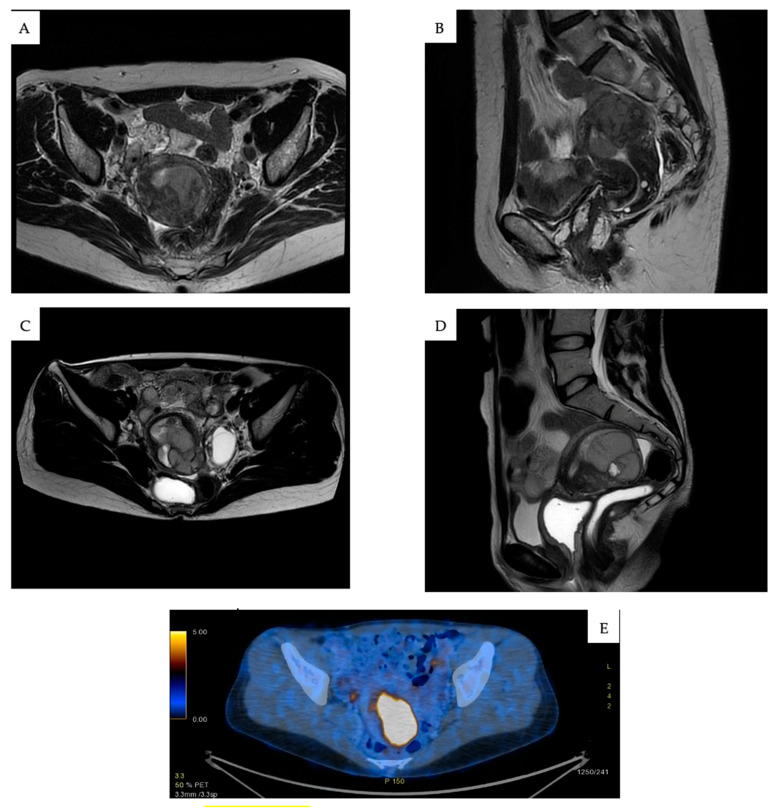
(A) Axial pelvic MRI image showing a hypointense endometrial mass with infiltrative margins in a patient with low-grade ESS (LG-ESS). (B) Sagittal pelvic MRI image highlighting endometrial thickening and myometrial infiltration in LG-ESS. (C) Axial MRI scan demonstrating extensive myometrial invasion and central necrosis in a case of high-grade ESS (HG-ESS). (D) Coronal MRI image depicting heterogeneous signal intensity with hemorrhagic and necrotic areas in HG-ESS. (E) PET-CT scan showing elevated FDG uptake in a metabolically active HG-ESS lesion. All figures are original and created by the authors.
